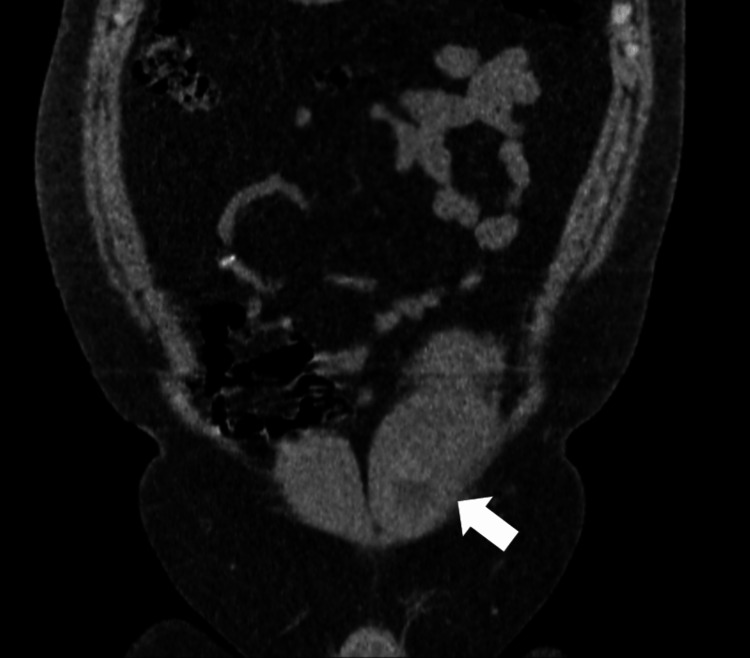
RSH located in the left rectus muscle. Coronal CT image (Patient 11)
